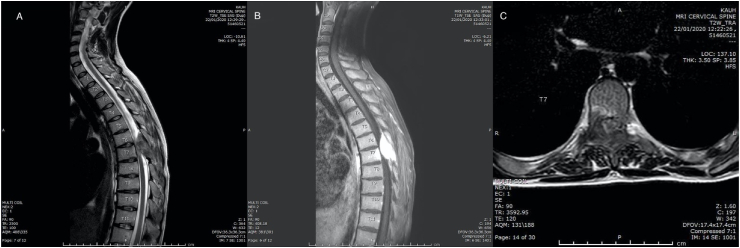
(A)(B) sagittal cervicothoracic MRI T2/T1 sequences showing large osteolytic bone lesion 3.5 × 1.8 cm, at level of T7. Significantly distorted the posterior elements of the corresponding vertebral spine. (C) axial MRI cut scan at level of T7 showing expansile osteolytic mass, involving the right pedicle and posterior arch, compressing the spinal canal and dislocating its content to the left.
